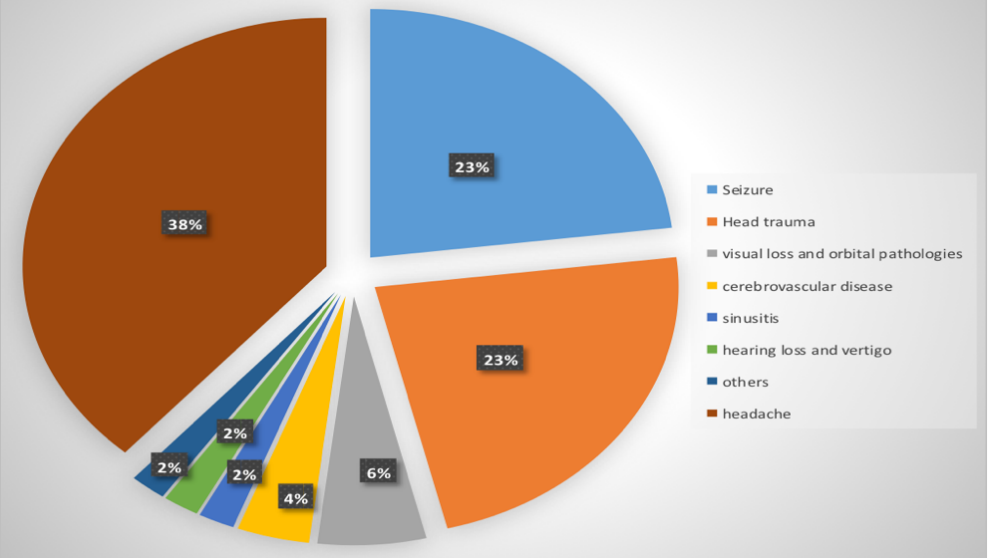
Clinical indications for Inappropriate Head CT scans
